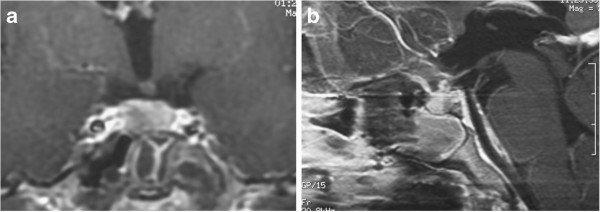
Case 1. Postoperative coronal (a) and sagittal (b) MR images revealing gross total removal of the tumor.
